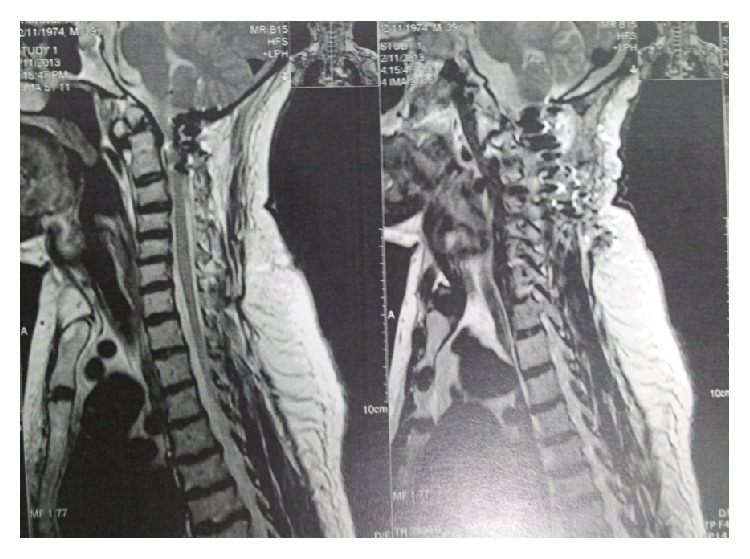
T1-weighted sagittal MR images showing alignment and decompression of craniocervical junction. Note short clivus, low Trochler Herophili, and small posterior fossa.
